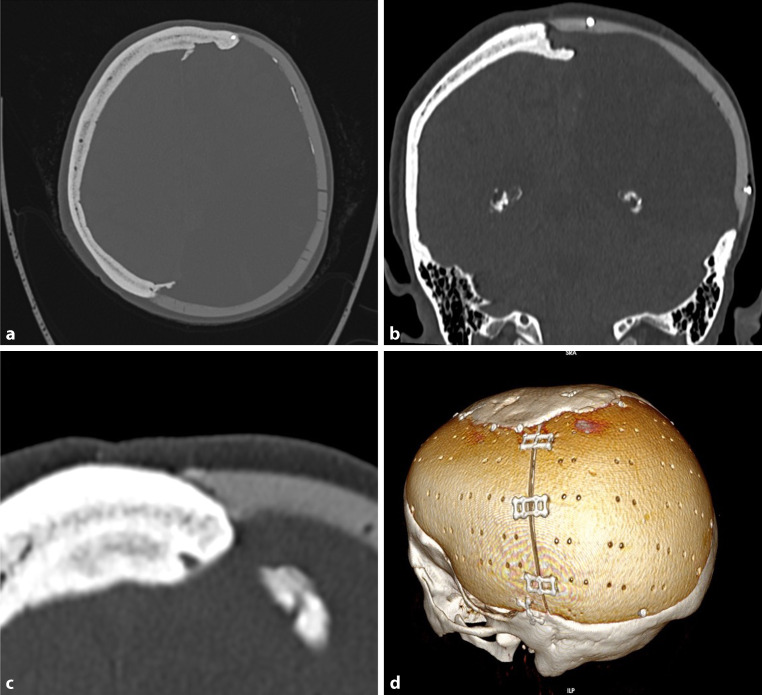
CT scans of reconstructed calvaria after meningeoma resection, 21 years after reconstruction; a Axial view, calcification of dura; b Coronal view, bone formation under the implant; c Bone-implant contact; d 3‑D image of the 2 implant pieces
